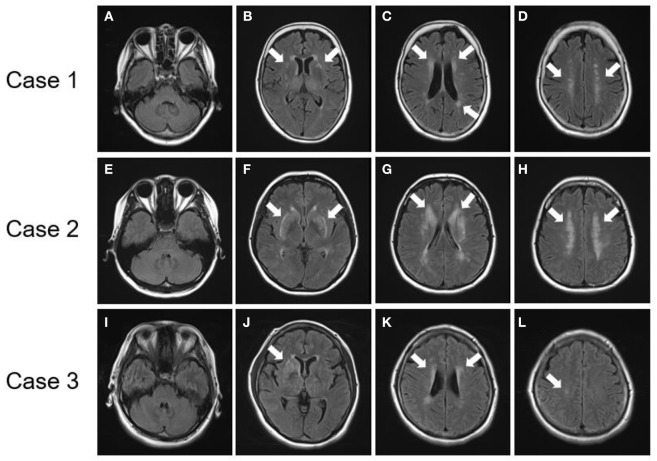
Brain MRI findings of patients with *NOTCH3* mutation. (A–D) FLAIR images of a patient with a *NOTCH3* p.R75P mutation (Case 1). (E–H) FLAIR images of a patient with a *NOTCH3* p.R75P mutation (Case 2). (I–L) FLAIR images of a patient with a *NOTCH3* p.R75P mutation (Case 3). (A,E,I) No patients presented lesions on their temporal pole lobes. (B,F,J) Arrows indicate hyperintensity lesions at external capsules. (C,D,G,H,K,L) Arrows indicate periventricular hyperintensity.
